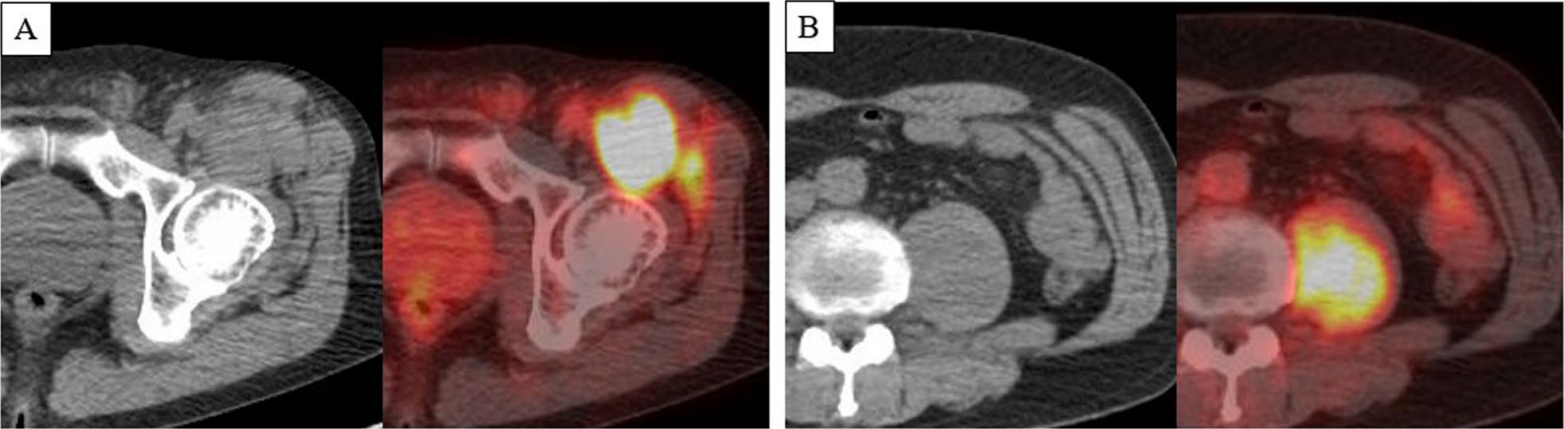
CT and FDG-PET/CT images of retroperitoneal dedifferentiated liposarcoma. FDG-PET/CT showed a SUVmax of 26.1 in the inguinal lesion (A) and a SUVmax of 17.8 in the iliopsoas muscle lesion (B)
